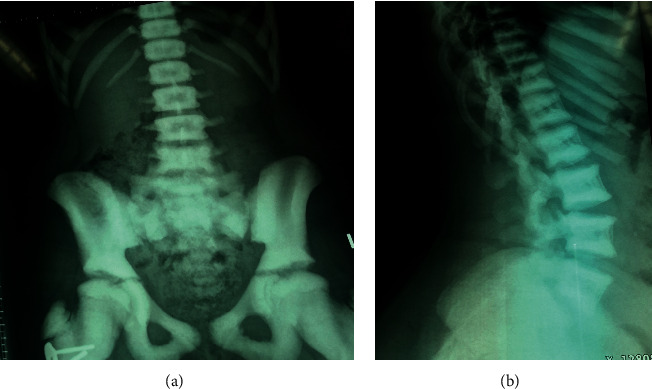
X-ray spine and pelvis showing dense vertebrae and pelvic bones (a), and Sandwich vertebrae (b).
